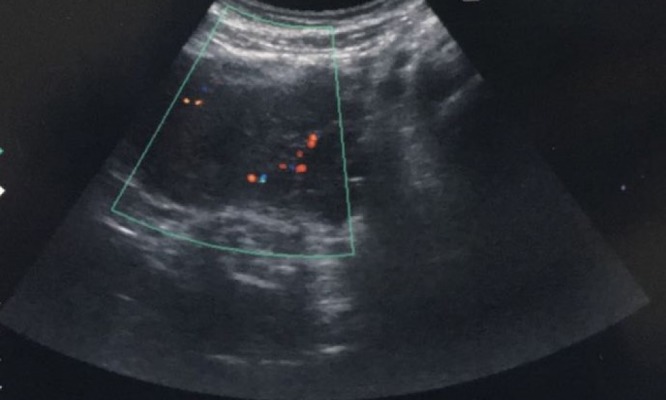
Follow up color Doppler ultrasound shows no evidence of blood flow within the pseudoaneurysm.
